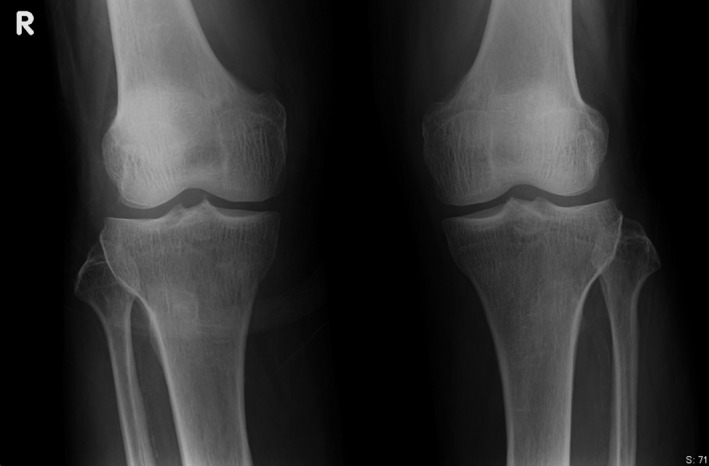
Radiograph of the knee joints. Knee radiographs showed soft tissue swelling but no bony or joint space abnormalities.
